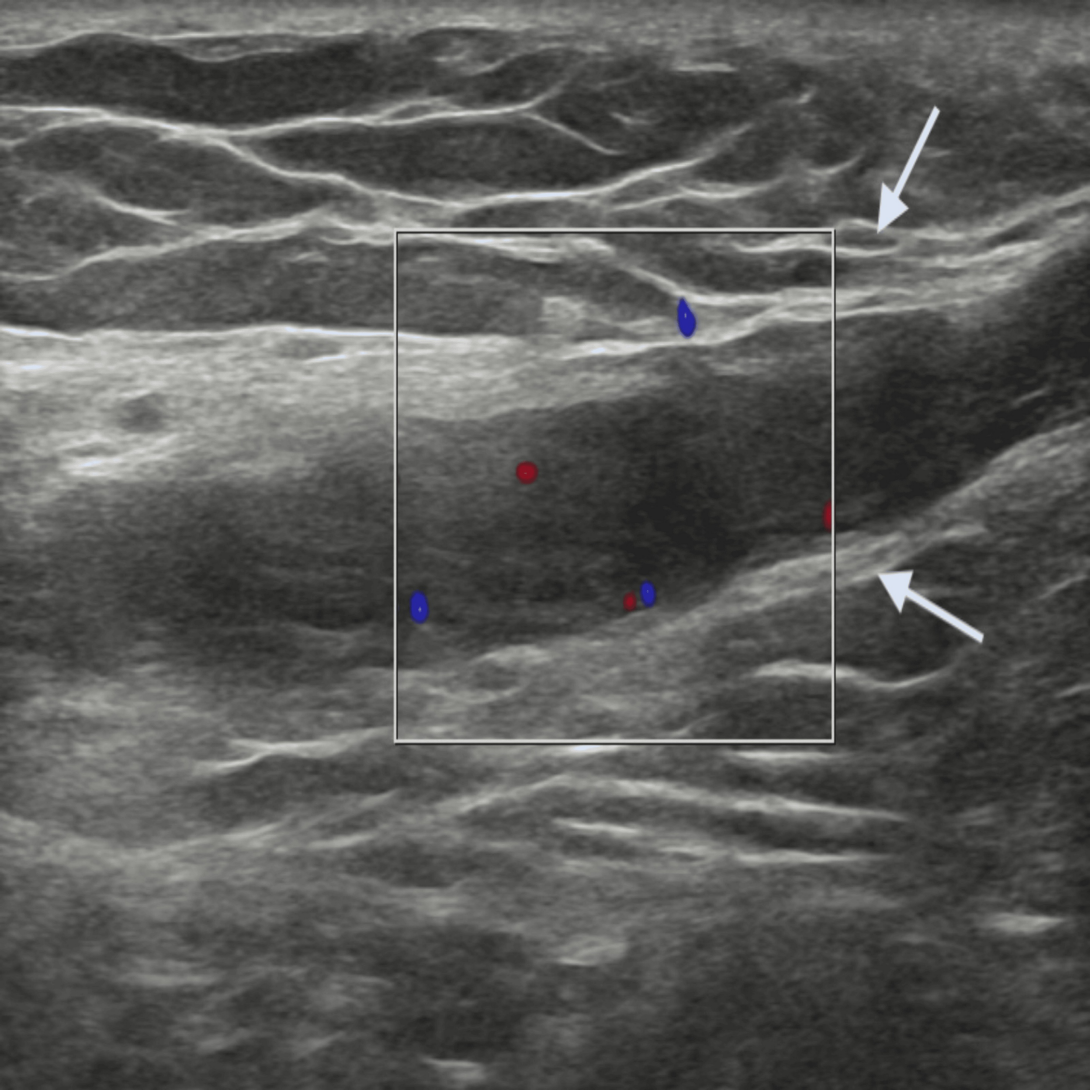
Color Doppler ultrasound of the right pampiniform venous plexus demonstrating absence of vascular flow within the thrombosed vein
